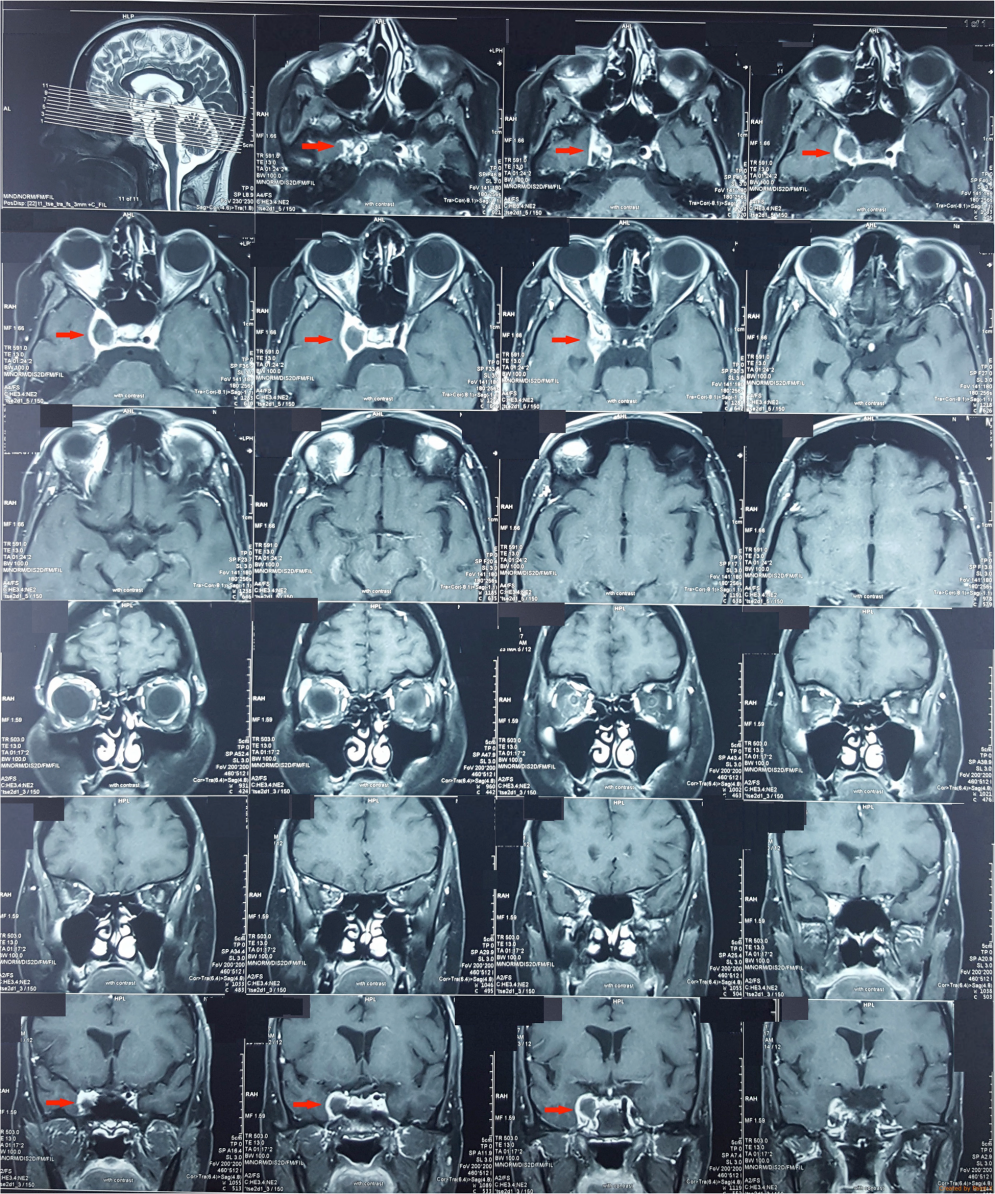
MRI showing dissection of the right internal carotid artery in the cavernous sinus (shown by the *red arrow*). A thrombosed false lumen of 1.5 × 1 cm, was seen. The thrombosed lumen was of intermediate signal intensity in and did not enhance with contrast. This was compressing the right cavernous sinus. Right internal carotid artery was significantly narrowed
